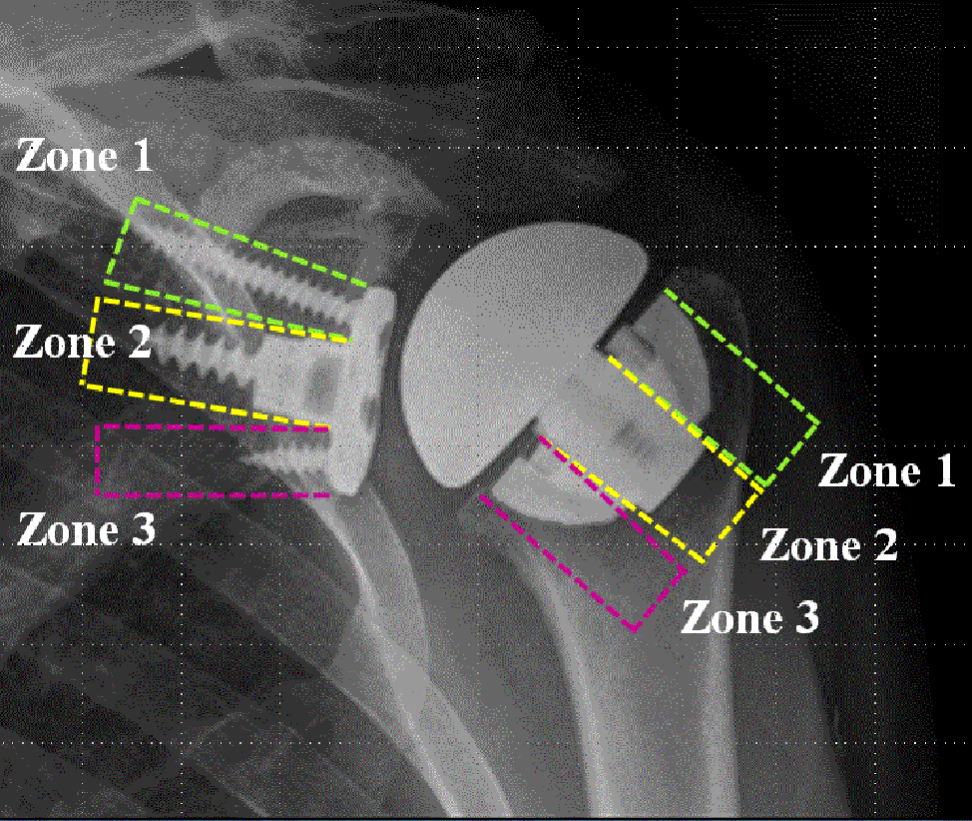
Radiolucent lines (RLL) for evaluation of loosening of the implant. To assess the RLL, implant-bone interface of glenoid and humerus are divided into three different zones in both anteroposterior view (AP) and axillary view of the plain radiograph. In glenoid, zone 1, zone 2 and zone 3 are defined as implant-bone interface around the superior (AP) or anterior (axillary view) peripheral screw, central screw, and inferior (AP) or posterior (axillary) peripheral screw, respectively. Similar to glenoid implant-bone interface, humeral implant-bone interface is evenly divided based on the peg of humeral tray
